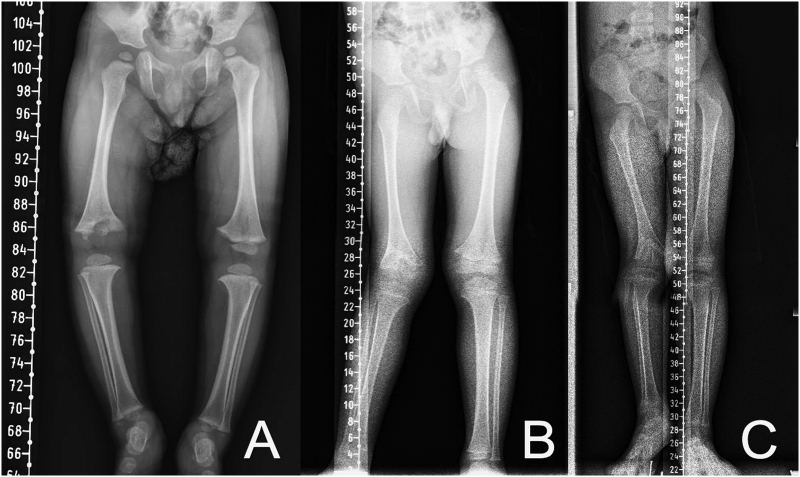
A. A 22-month-old boy radiograph long leg stitch view X-ray evaluation with O-shaped lower extremity. B. 10 months later the radiograph evaluation showed the lower extremity became X-shaped. C. 14 months later there was a 3 cm limb-length discrepancy.
